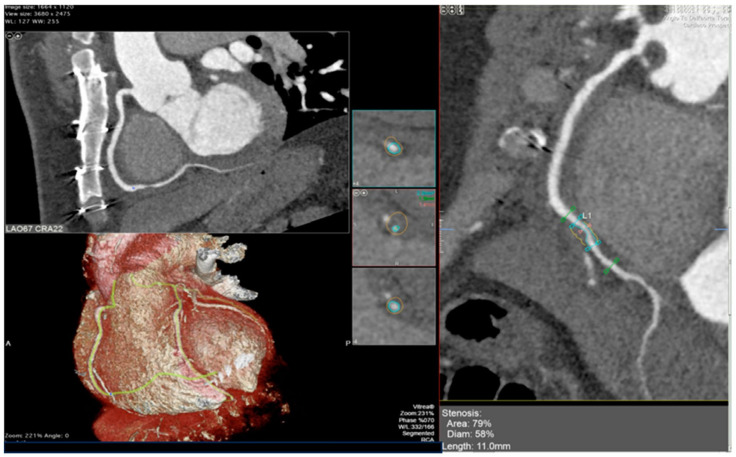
CCTA reveals grade 3 CAV based on ISHLT criteria. A calcified plaque is identified in the second segment of the right coronary artery, shown in the reconstructed view on the right panel and corresponding orthogonal views on the left panel. Adapted from Pergola et al., 2023 [62]. CCTA—Cardiac Computed Tomography Angiography; CAV—Cardiac allograft vasculopathy; ISHLT—International Society for Heart and Lung Transplantation.
